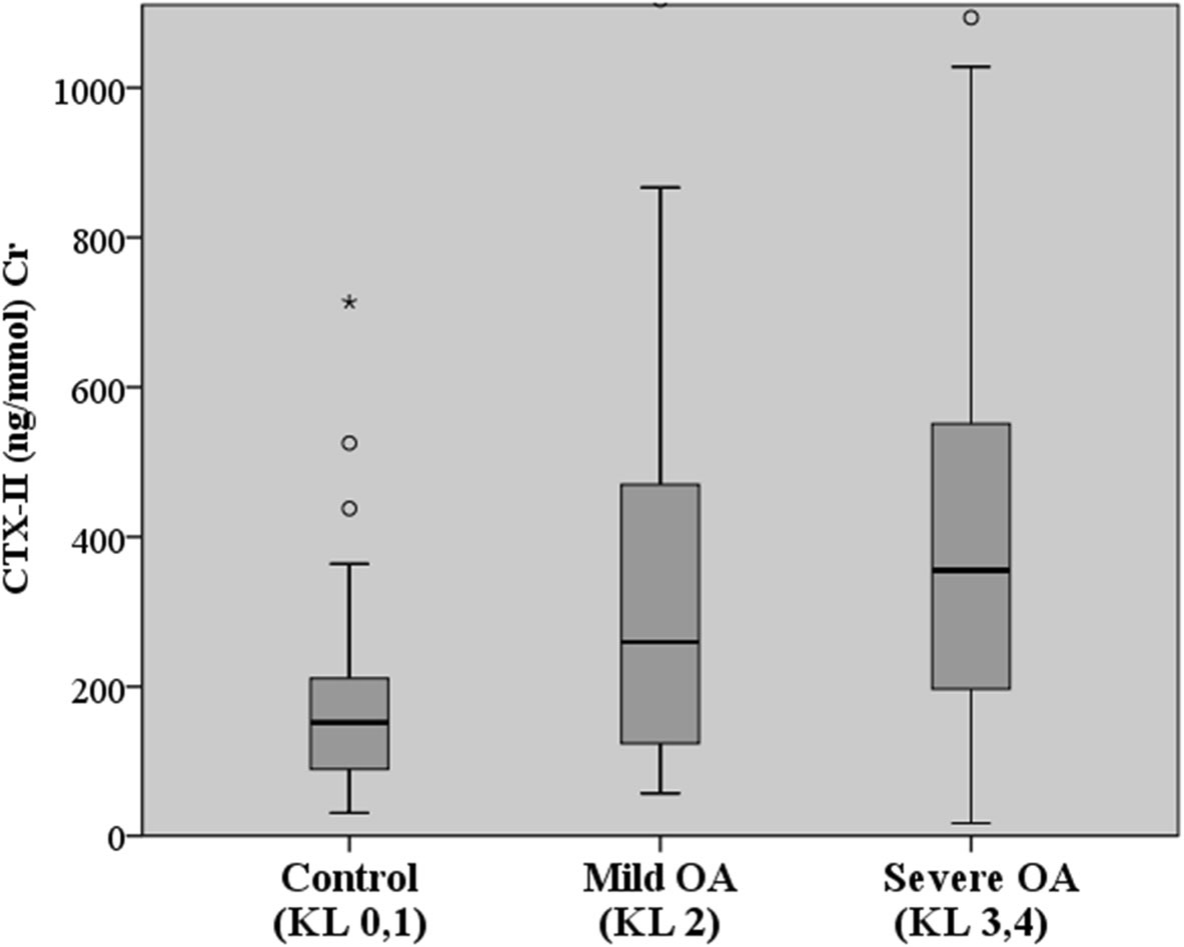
Comparison of the levels of urinary CTX-II in different radiographic knee severities
